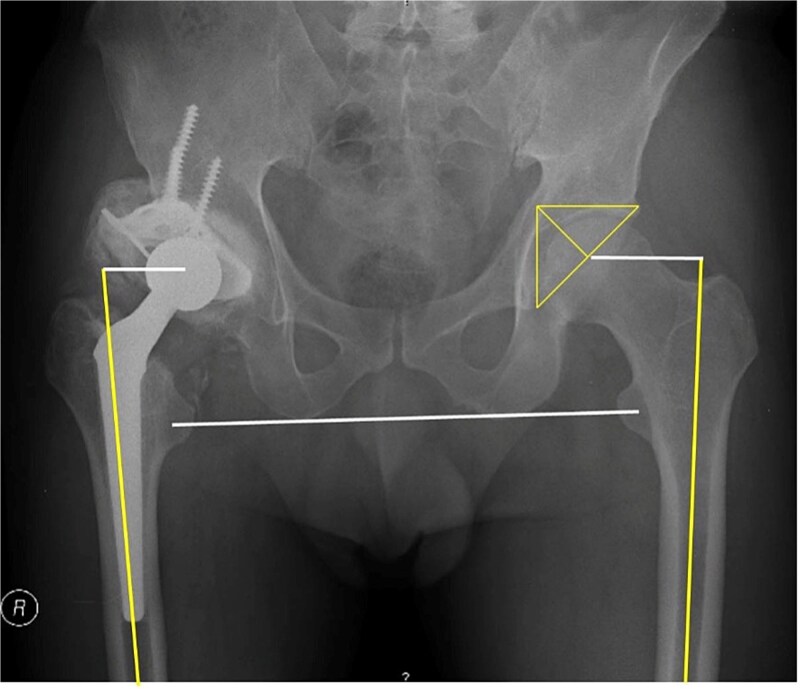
Postoperative THA radiograph at 5-year follow-up demonstrating stable implant fixation, absence of bone graft resorption, and acceptable restoration of the hip’s rotation centre and offsets. Correct inclination of the cemented polyethylene cup and near-equalisation of limb length are maintained.
